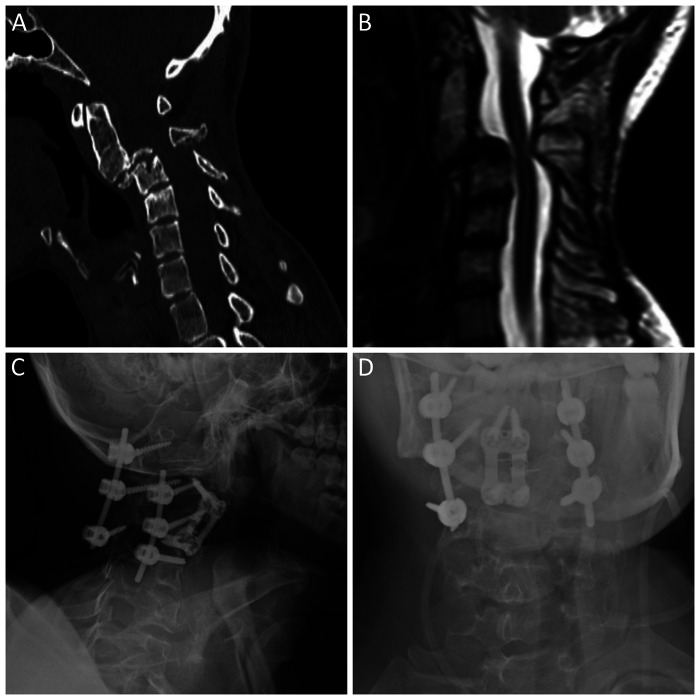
Preoperative imaging and postoperative radiographs demonstrating severe cervical instability and staged 360-degree stabilization. In December 2020, a preoperative sagittal computed tomography (CT) scan (A) demonstrated severe C2–C3 anterolisthesis with marked C3 vertebral body collapse, and (B) a sagittal T2-weighted MRI demonstrated marked spinal canal narrowing at the C2–C3 level. Postoperative radiographs demonstrate (C) a lateral view showing anterior C3 corpectomy with C2–C4 cage reconstruction and anterior plate fixation combined with posterior screw–rod instrumentation spanning C1–C4, and (D) an anteroposterior view confirming the combined anterior C2–C4 construct and posterior instrumentation.
